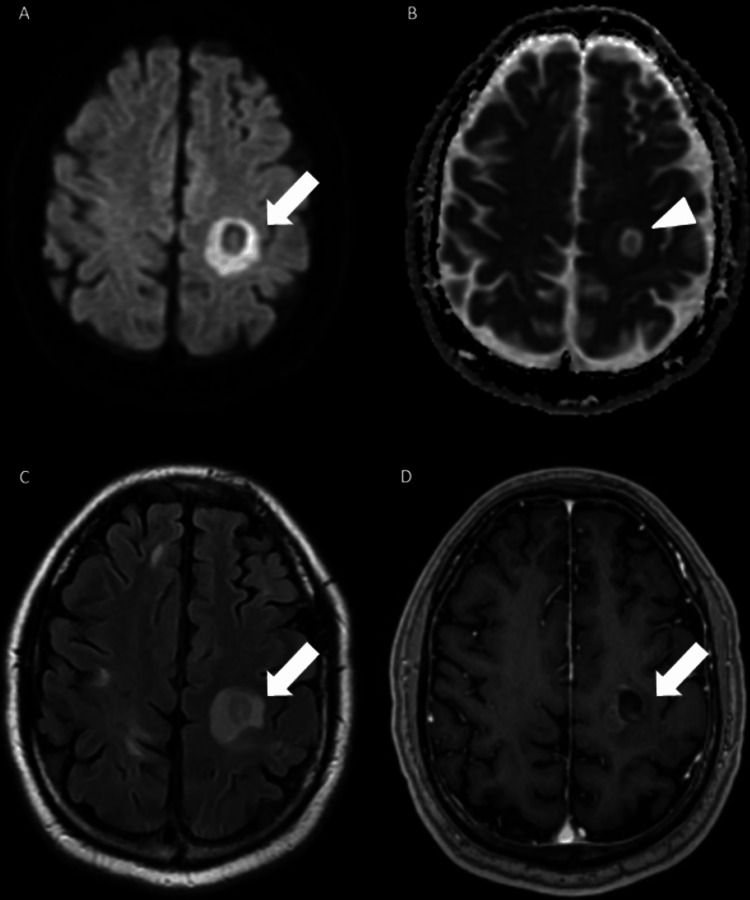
Axial MRI brain sequences. The lesion corresponding to the CT hypodensity demonstrates diffusion restriction on DWI (A), T2 hyperintensity (C), and heterogeneous open-ring enhancement on post-contrast T1-weighted imaging (D), all marked by arrows. On the ADC map (B), a layered “onion peel” appearance is observed (arrowhead), which is faintly discernible on other sequences. No significant mass effect is present despite the lesion’s size. DWI: diffusion-weighted imaging; ADC: apparent diffusion coefficient.
